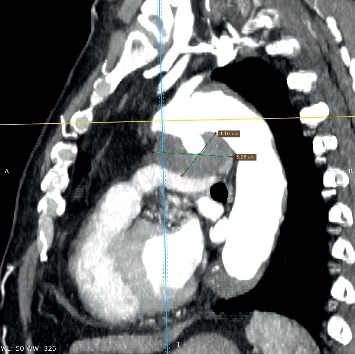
Computed tomography angiography scan of an older man with partially thrombosed PDA aneurysm (own material).
